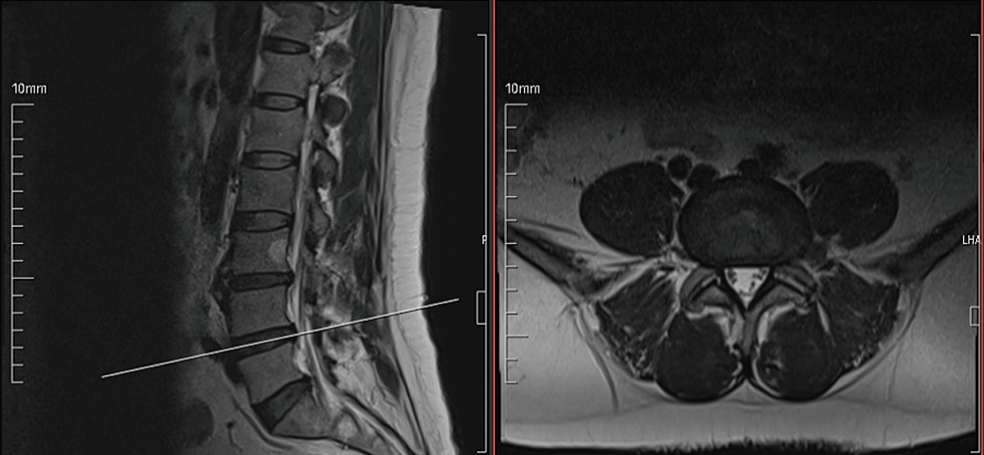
Magnetic resonance images Sagittal (left-hand side image) and axial (right-hand side image) images of left-sided far lateral lumbar disc herniation at the L4-5 level.
